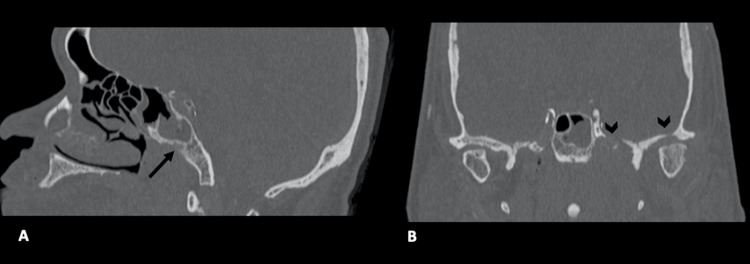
HRCT of temporal (A) in sagittal and (B) coronal views three months later show persistent fullness in the posterior nasopharynx with clival erosion (long arrow). There are also worsening bony erosions at the left base of the skull (arrowhead). HRCT: high-resolution computed tomography
